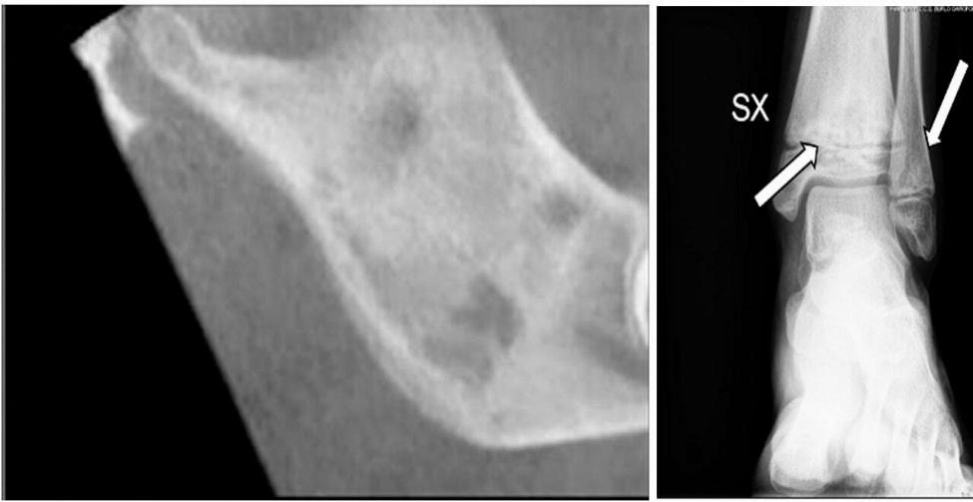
CT cone beam: inhomogeneous bone thickening of the branch and of the right mandibular angle associated with erosive areoles (a); left ankle X-Ray with osteolytic lesion of the tibial metaphysis (b)
